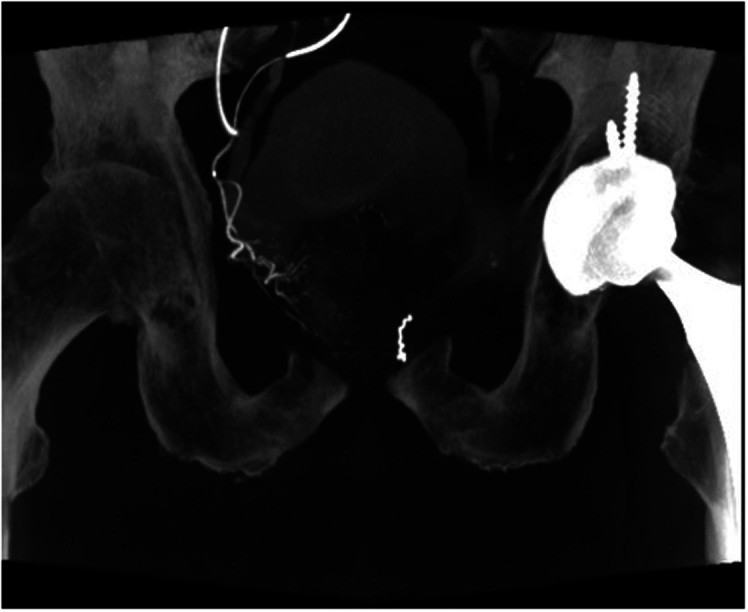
Three-dimensional cone beam computed tomography with contrast injection in the right prostate artery confirming gland enhancement (left side already treated with a protective coil placed at the base of the left lobe).
